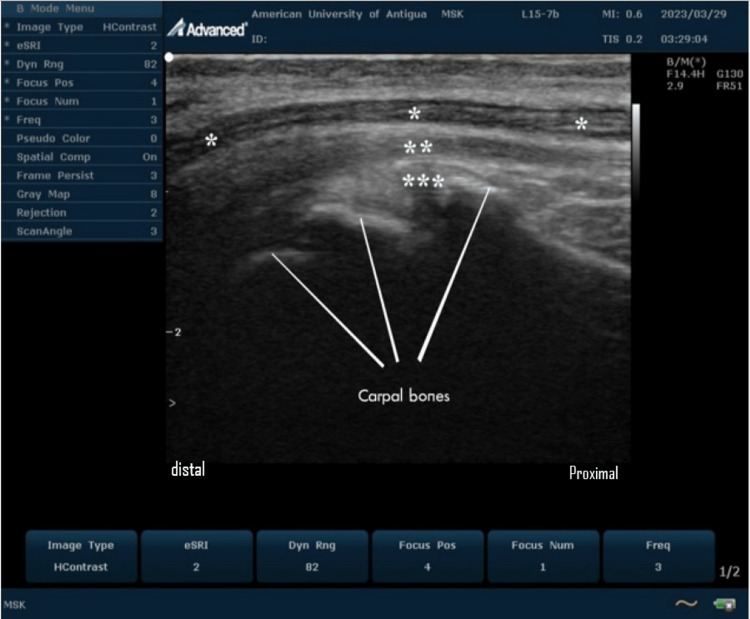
Ultrasound imaging of the normal carpal tunnel at the volar aspect of the wrist On this longitudinal scan, the median nerve (*) is seen overlying the relatively hyperechoic superficial () and deep (*) flexor tendons of the wrist. The median nerve appears as a hypoechoic honeycomb fibrillar structure. This is an original image from the ultrasound lab department of the American University of Antigua
